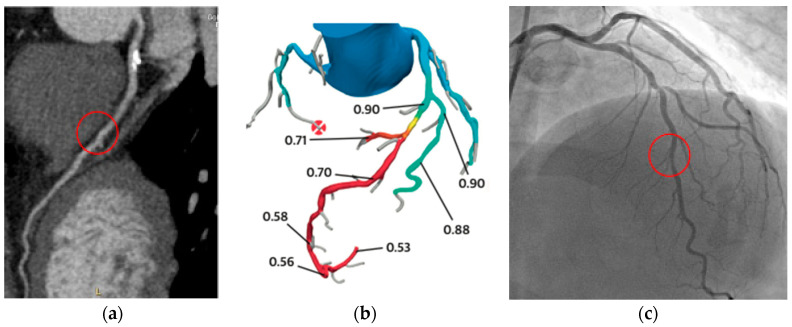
S.M. 54 years-old, male; dyslipidaemia, smoker, PAD; breath shortness; CCTA (a): moderate mid-LAD stenosis (see also Figure 2); FFR-CT was performed (b): pressure drop after diagonal branch; ICA (c): significant tandem-stenosis of LAD (left anterior descending artery). CCTA: coronary computed tomography angiography; LAD: left anterior descending artery; FFR-CT: fractional flow reserve derived from coronary computed tomography; ICA: invasive coronary angiography.
